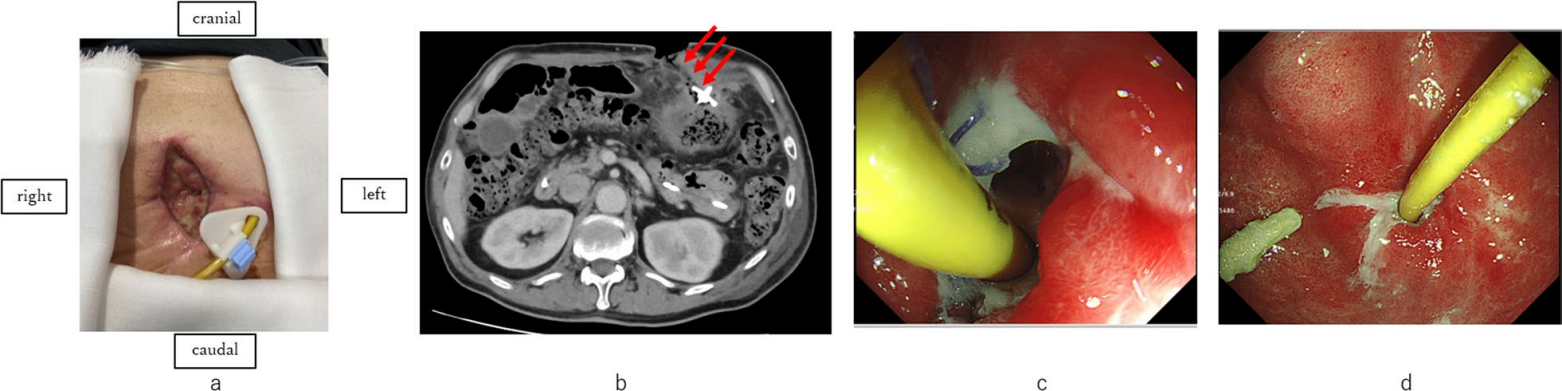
Postoperative findings. a Wound dehiscence occurred, and food residue exuded from a gastrocutaneous fistula. b CT showing a gastrocutaneous fistula (red arrows). There was no sign of free air or ascites. c EGD revealed a gap around the PEG-J. d A follow-up EGD showed the disappearance of the gap around the PEG-J.PEG-J, percutaneous endoscopic gastrostomy with a jejunal extension tube; *EGD* esophagogastroduodenoscopy; *CT* computed tomography
